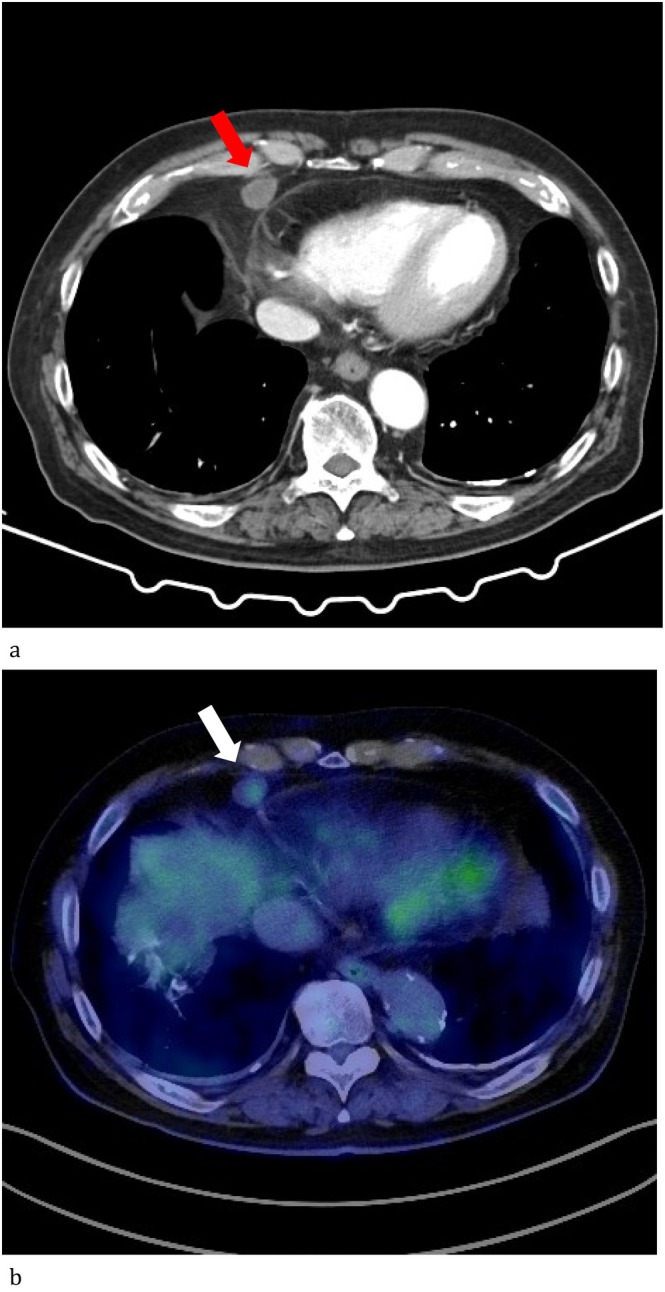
(a) Chest CT. An enlarged right cardiophrenic angle lymph node is seen (orange arrow). (b) Positron emission tomography. A slight fluorodeoxygrucose accumulation was observed in the tumour (white arrow).
